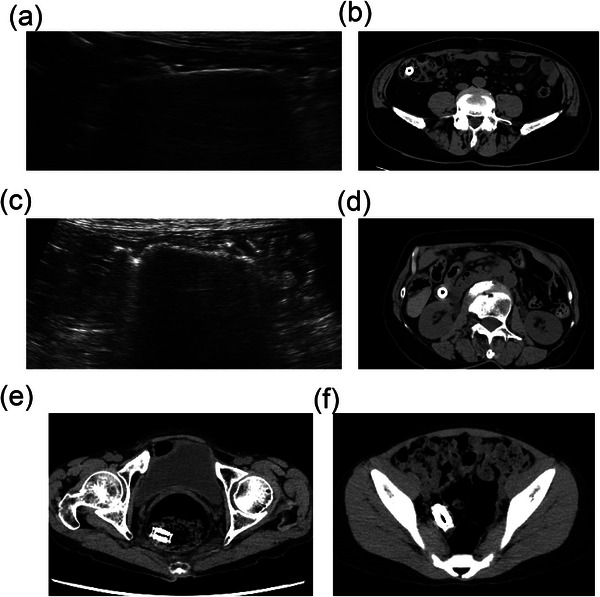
PC location identification by ultrasound and CT images. (a, b). Images of PC in the large intestine. PC was identified in the ascending colon, extending from the ileocecal junction. (c, d). Images of PC in the small intestine. PC was identified in the small intestine, where Kerckring folds were observed. (e) A case of PC buried in stool in the rectum, making it difficult to identify by ultrasound. (f) A case of PC located deep in the dorsal portion of the sigmoid colon, making it difficult to identify by ultrasound. PC, patency capsule; CT, computed tomography.
